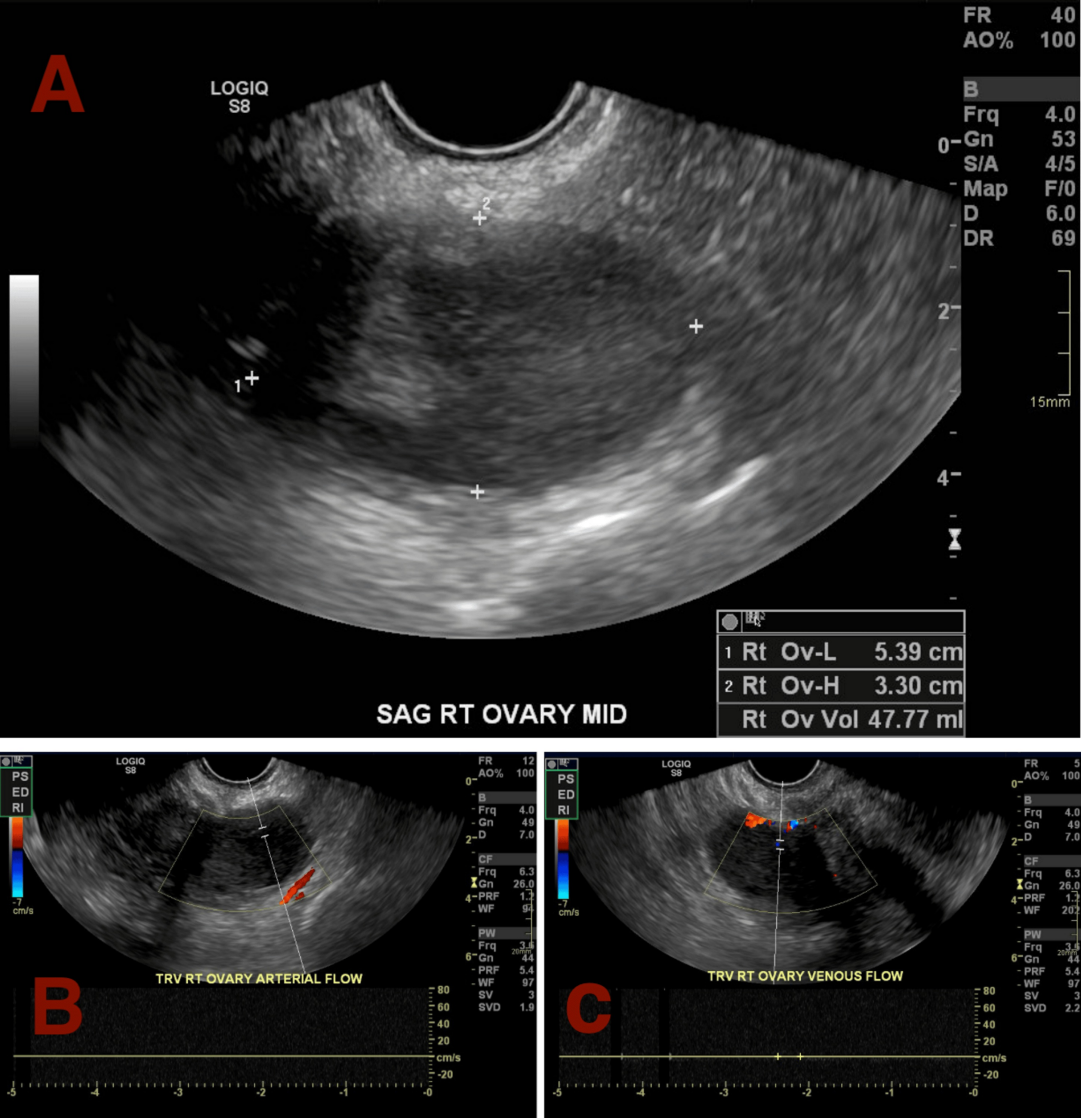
Ultrasound depicting enlarged size for the right ovary (A), measuring at 4.6 × 2.9 × 2.9 cm, followed by Doppler ultrasound depicting loss of arterial (B) and venous (C) flow to the right ovary for Patient A.
